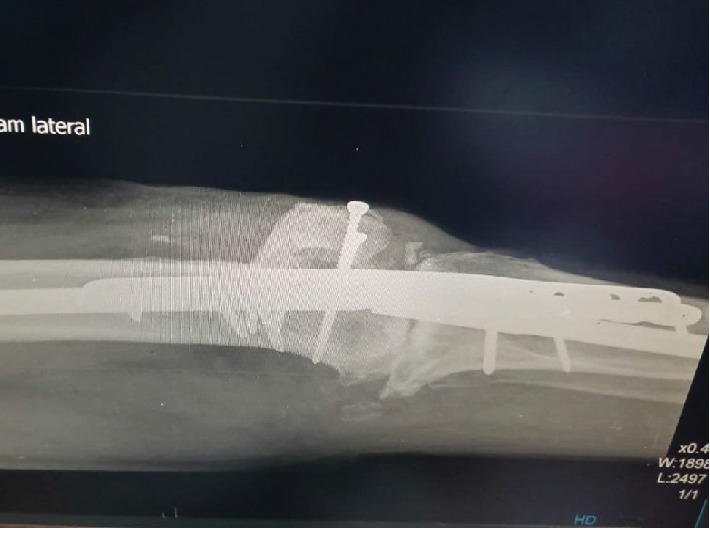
Radiograph shows knee fusion in lateral view.
